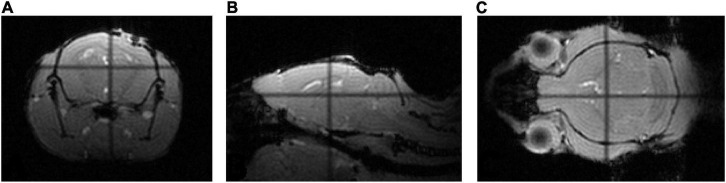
MRI localizer images. (A–C) Coronal, sagittal, and axial GE images acquired through a localizer scan show little to no distortion around the brain.
